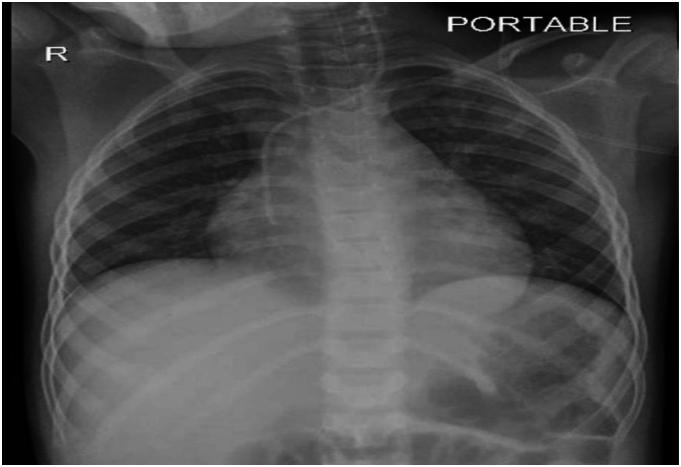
Chest x-ray showing dramatic improvement of lungs parenchyma.
